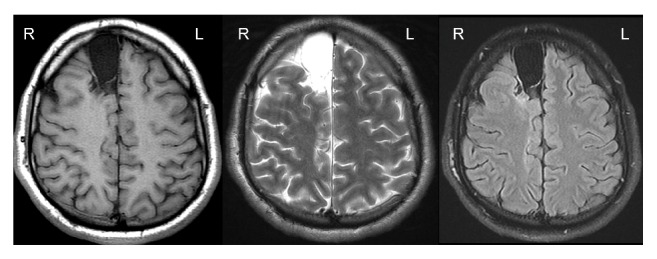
Transaxial multisequential magnetic resonance imaging of the brain in the form of a T1 weighted image (WI), T2 WI, and fluid-attenuated inversion recovery (FLAIR) WI, showing an extra-axial, well-defined elliptical lesion located in the right frontal region. The lesion follows the signal intensity of the CSF in all sequences.
